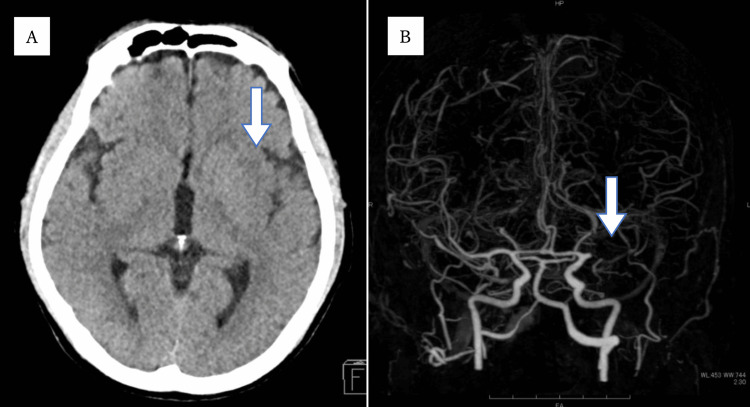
Initial CT scan and CT angiography Head CT showed early signs of ischemic infarction in the left insular cortex (A: arrow). Head CT angiography indicated occlusion in the horizontal part of the left middle cerebral artery (B: arrow).
